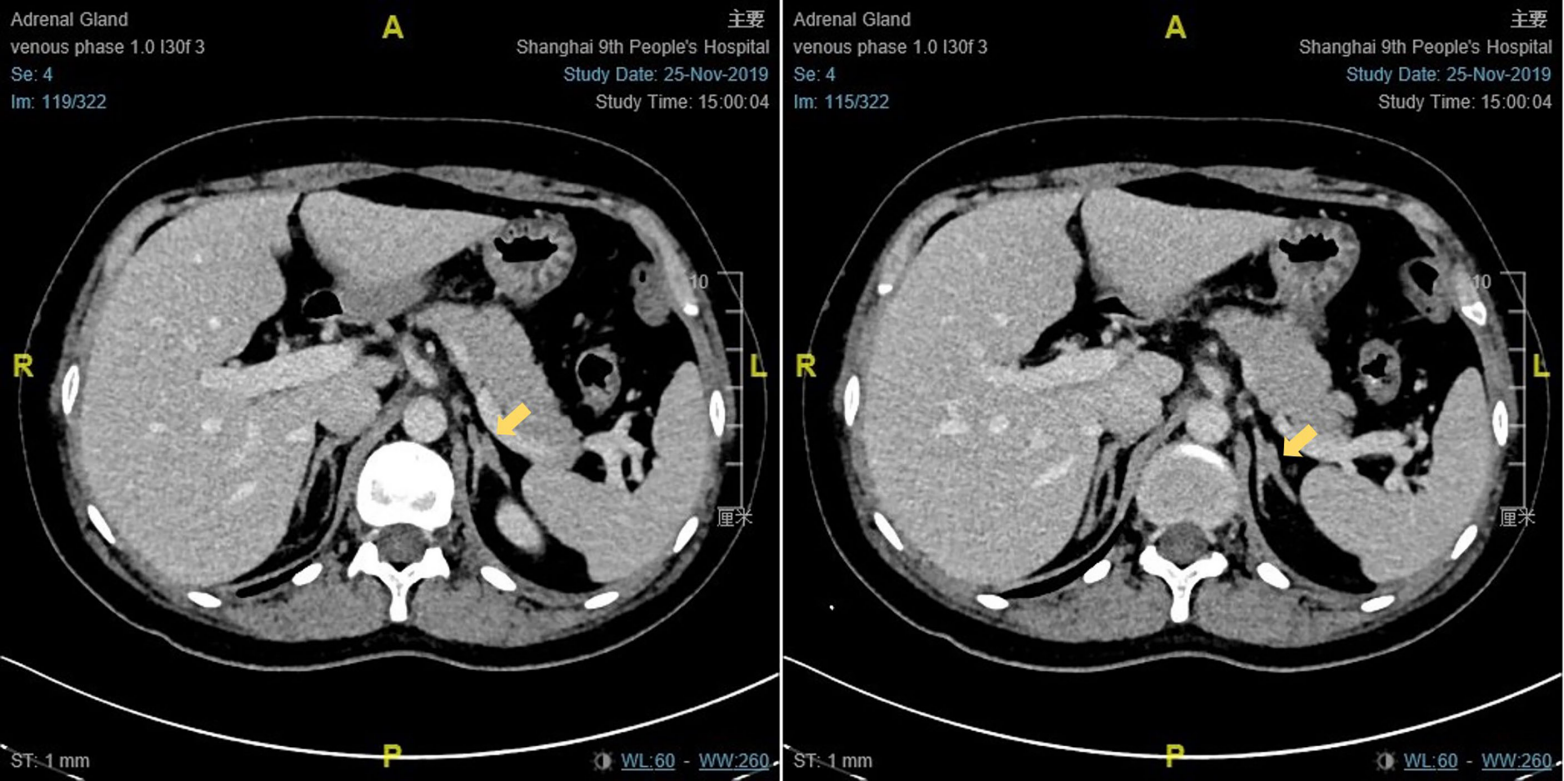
The enhanced CT scanning result of proband 1.
